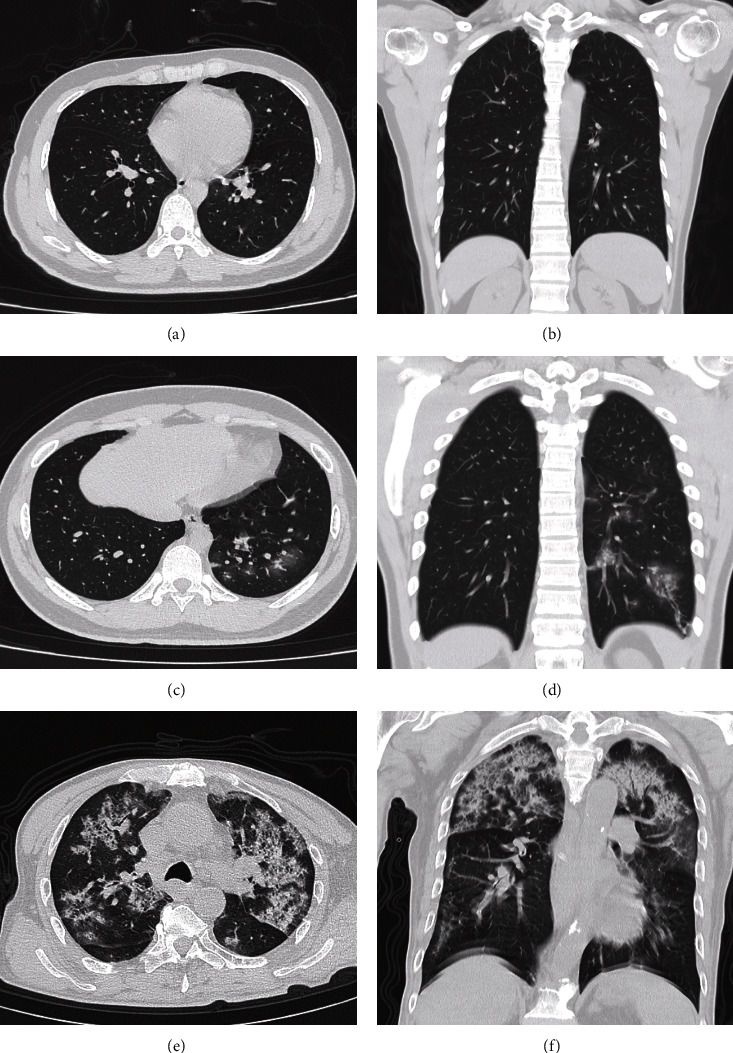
Examples of the pneumonia risk model applications. (a and b): A 32-year-old male presented with a 1-day history of fever with a maximum temperature of 39.2°C. At the time of presentation, he was confirmed positive by nucleic acid testing for SARS-CoV-2. His routine blood test showed a CPR of 14.08 (InCRP = 2.70) and %Mon of 26.50. Considering his age of 32, the patient got a total of 75 points according to our pneumonia risk prediction model, with a pneumonia risk prediction probability of <0.1. The patient underwent a CT chest scan, which showed no abnormal findings. (c and d): Male, 17 years old, presented 4 days ago with a fever with a maximum temperature of 39.0°C. On presentation, he was confirmed positive by nucleic acid testing for SRRS-CoV-2. His routine blood test showed a CPR of 82.45 (InCRP = 4.41) with a %Mon of 8.30. Considering his age of 17, the patient had total points of 152 according to our pneumonia risk prediction model, with a pneumonia risk prediction probability of 0.68. The patient underwent a chest CT, which showed multiple lamellar ground-glass opacities in the lower lobe of the left lung, with a peripheral distribution and thickened blood vessels within the lesion. (e and f): A 63-year-old male with a 1-week history of malaise was confirmed to be nucleic acid test positive for SARS-CoV-2 on presentation. His routine blood test showed a CRP of 259.68 (InCRP = 5.56) with a %Mon of 5.00. Considering his age of 63, this patient had total points of 192 according to our pneumonia risk prediction model, with a pneumonia risk prediction probability of >0.9. The patient underwent a chest CT, which showed multiple lamellar hyperintensities in multiple lobes of both lungs with solid lesion density, bronchial air sign within, and halo sign at the edges of some lesions.
